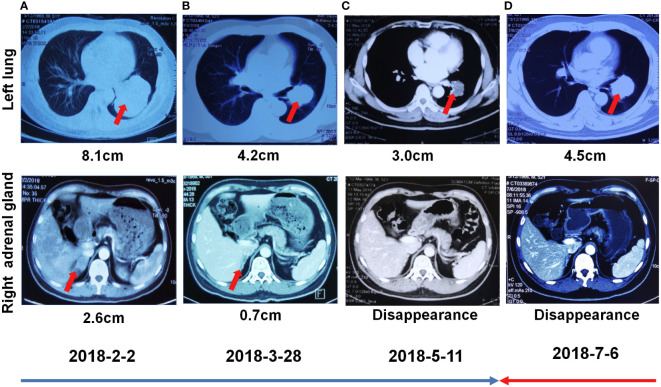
First-line treatment with crizotinib. (A) Chest CT showed focal changes in the left lung and right kidney on February 02, 2018. (B) Chest CT showed focal changes in the left lung and right kidney on March 28, 2018. (C) Chest CT showed focal changes in the left lung and right adrenal gland on May 11, 2018. (D) Chest CT showed focal changes in the left lung and right kidney on July 6, 2018.
